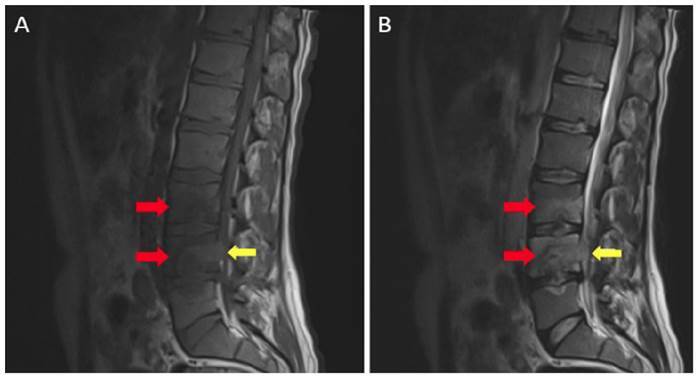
Noncontrast sagittal T1-wighted (T1W) (A) and T2-weighted (T2W) (B) lumbar magnetic resonance (MR) images show cortical irregularities accompanied by peripheral medullary bone marrow edema (red arrows) in the end plates adjacent to the L3-L4 and L4-L5 intervertebral discs. In addition, there are heterogeneous T2 signal increases in defined intervertebral discs. An abscess collection narrowing the spinal canal is also observed adjacent to the posterior corpus of the L4 vertebra (yellow arrows).
